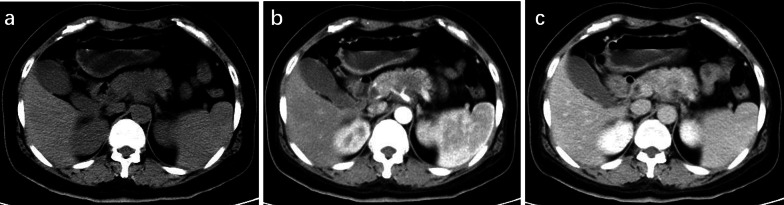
PDAC of the pancreatic body in a 51-year-old woman. a Unenhanced CT scan shows an ill-defined solid mass with a size of 4 cm. The CT value of the tumor is 36 HU. b Arterial phase CT scan shows diffusely mild homogeneous enhancement of the lesion and the celiac trunk is involved. The CT value of the tumor is 45 HU. c Portal phase CT scan show s progressively filling enhancement. The CT value of the tumor is 61 HU
